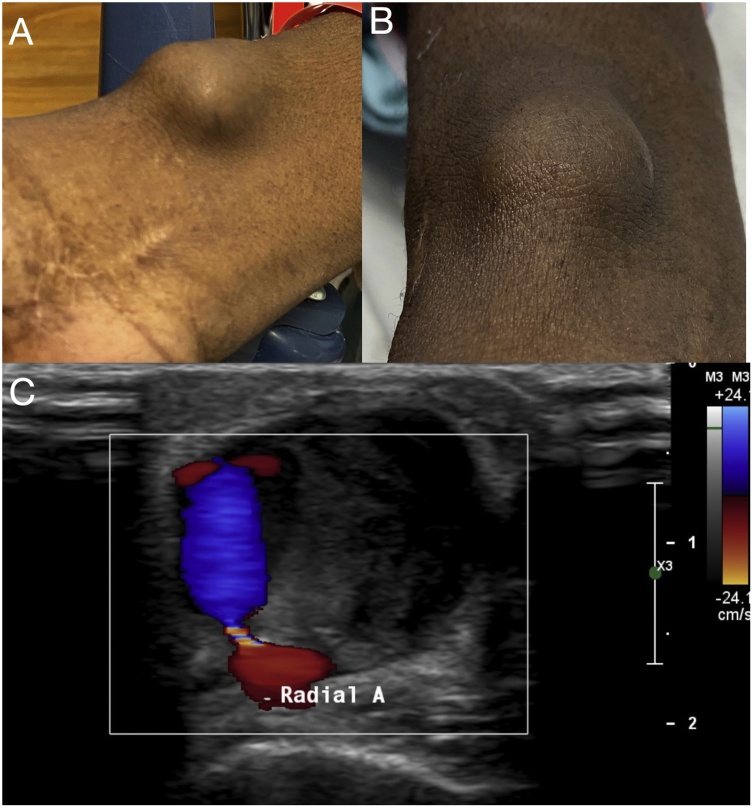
A & B: Visual inspection of radial aetery pseudoaneurysm. C: Duplex Ultrasound of radial artery with partially thrombosed, large pseudoaneurysm measuring at 3.2 × 1.8 cm and a 1.3 cm neck.
